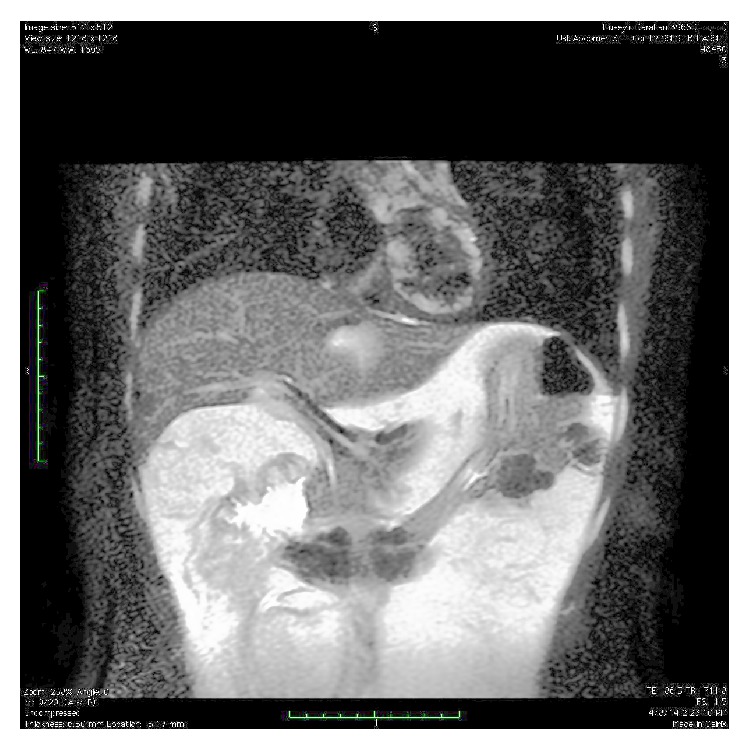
Coronal T2 weighted MR imaging of diffuse abdominal lipomatosis, luminal narrowing at distal part of stomach and bulb secondary to diffuse lipomatosis and antral submucosal lipoma with deplased intestinal loops.
